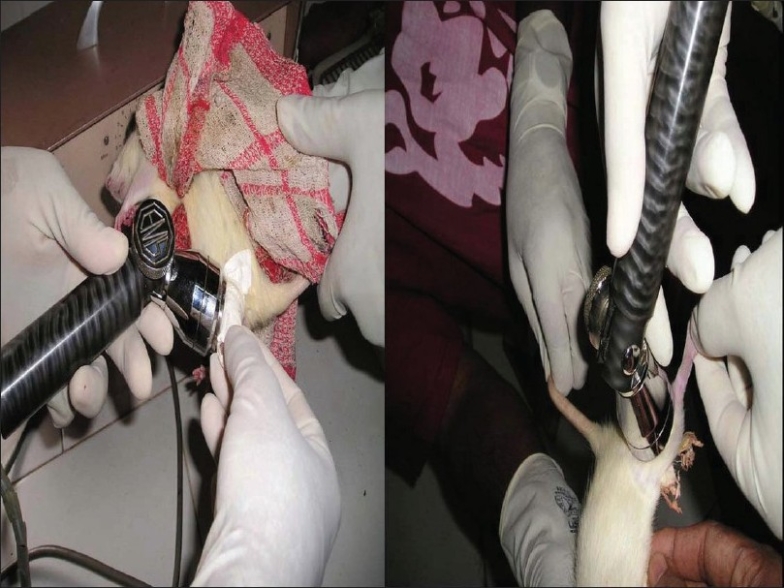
Therapeutic ultrasound in test group
